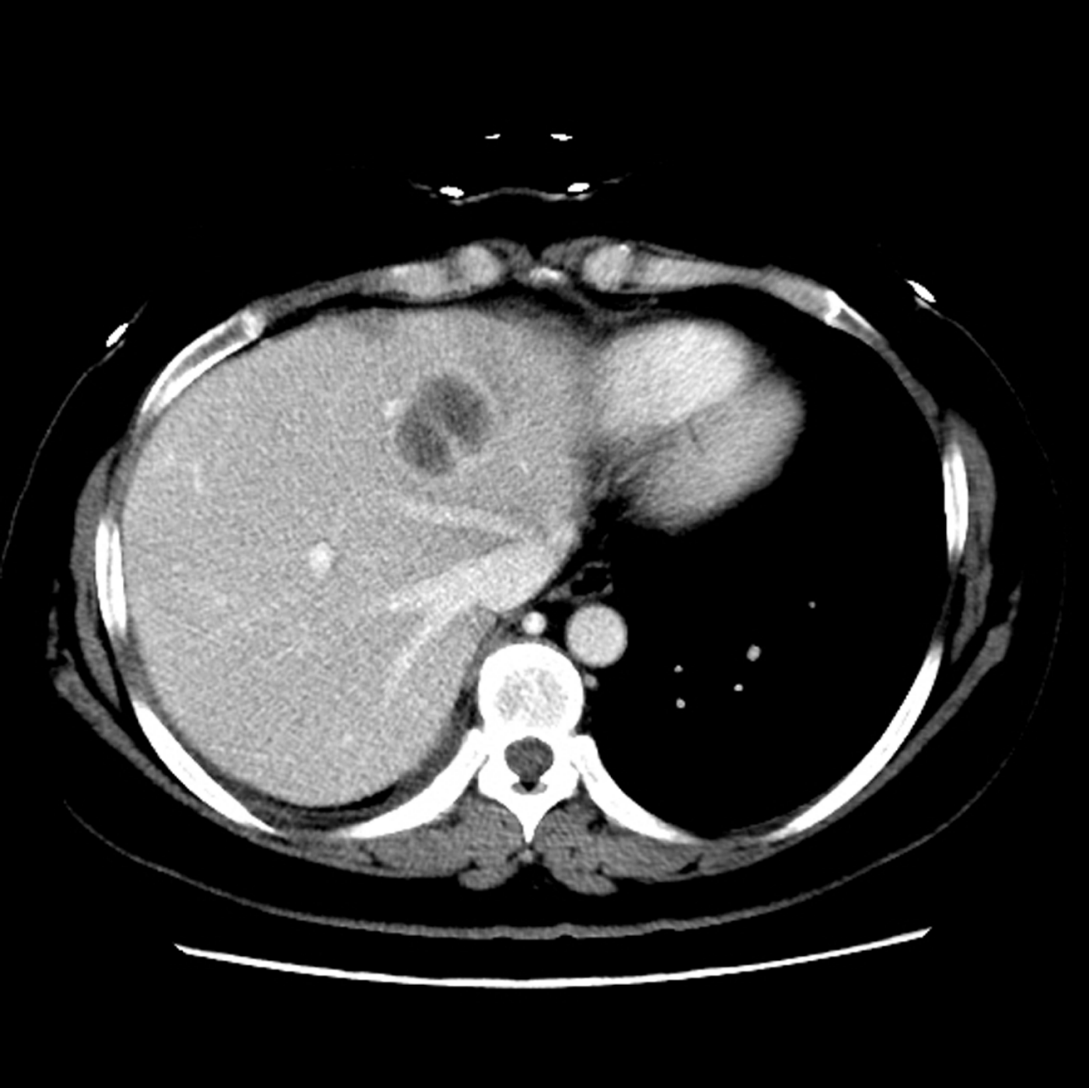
Computed tomography scan images showed new hepatic lesions appeared after 6 months of AZD9291 treatment
